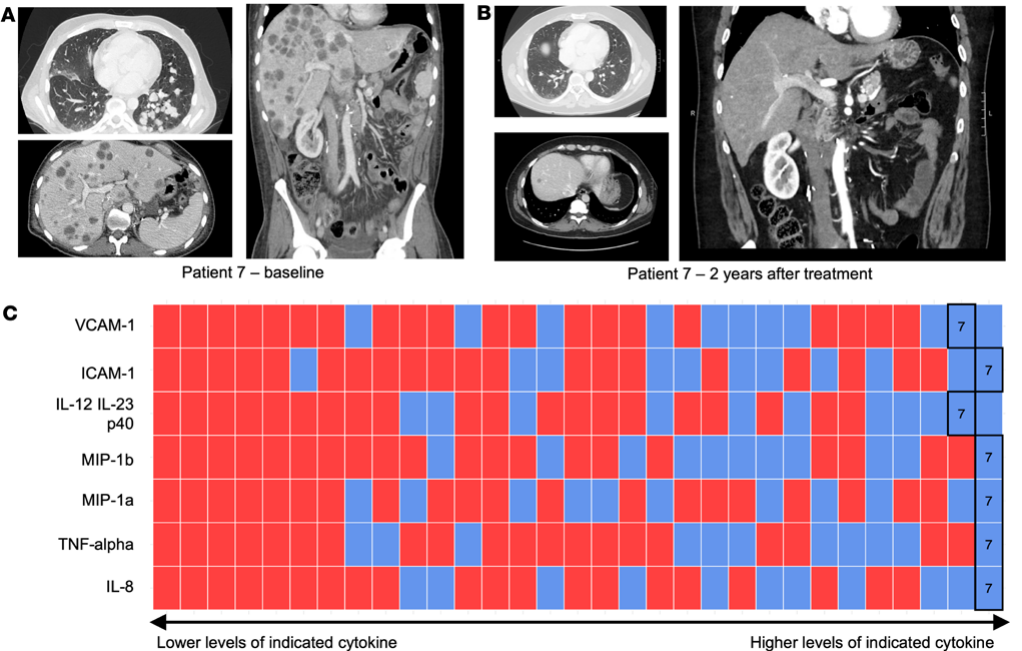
Relative cytokine levels in patient with exceptional response to ICI. (A and B) CT scan images of exceptional responder patient 7 with innumerable metastases in lungs and liver at baseline (A) and 2 years after treatment (B). (C) Levels of key cytokines in patients 7, 20, and 29 (3 patients with sarcomatoid/rhabdoid features) relative to those of other patients. Within each row: every patient is represented by a bar, ordered left to right by increasing level of indicated cytokine and color coded by clinical response (blue, PR/CR; red, PD/SD). Relative position of patient 7 is labeled “7”.
